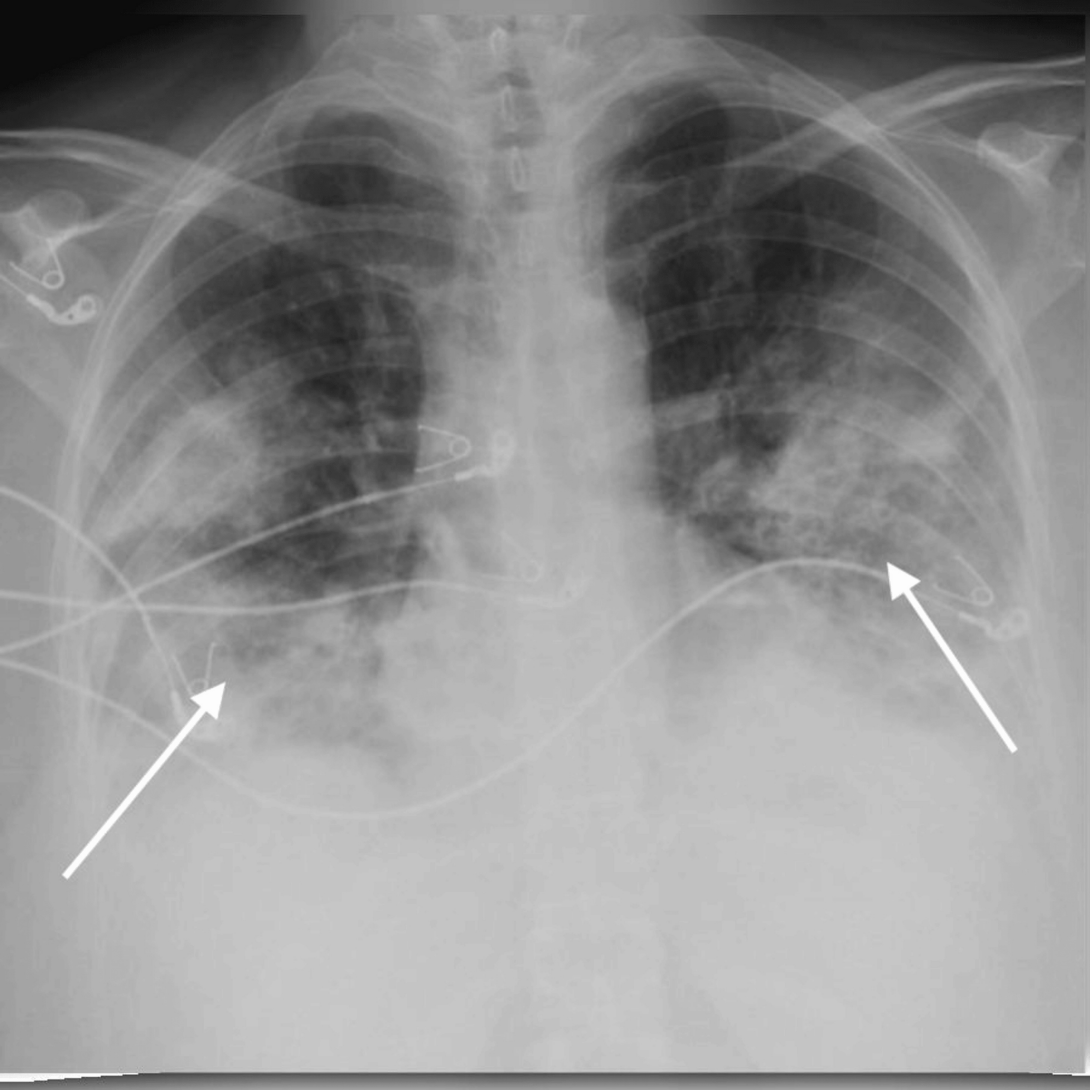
Chest X-ray 24 hours after admission
